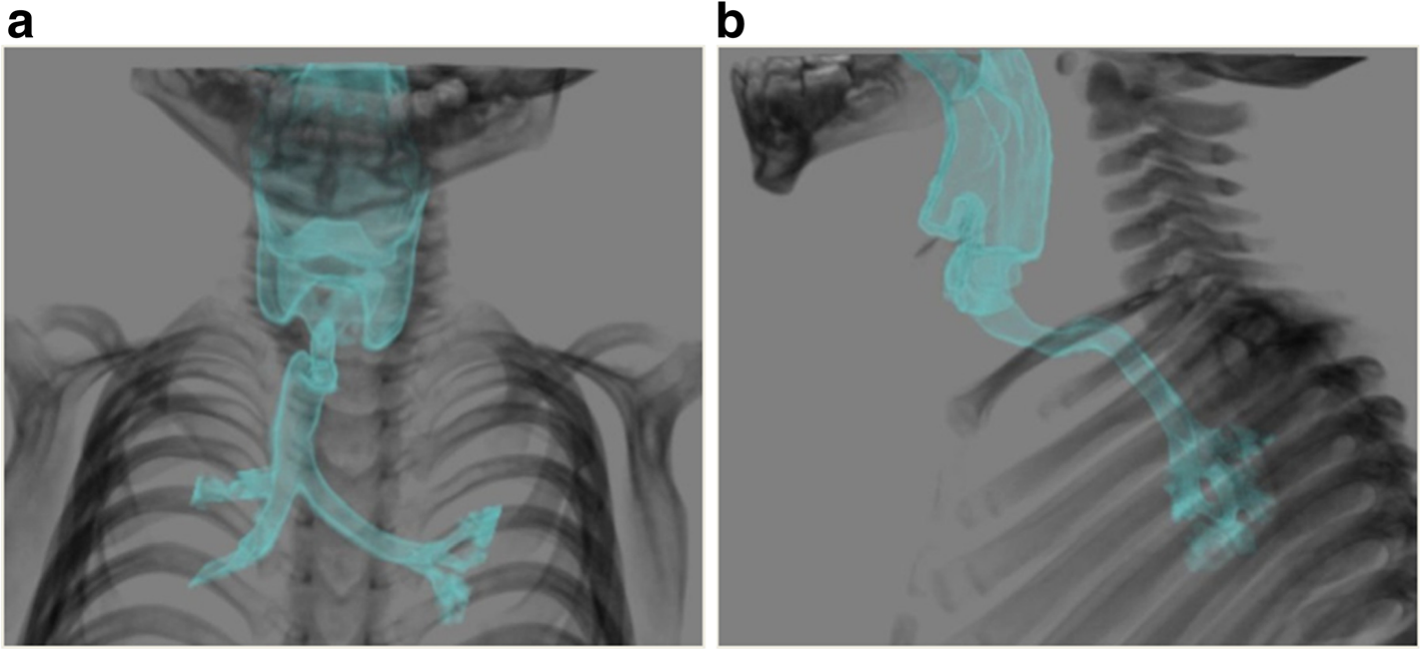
Computed tomography before operation. a Frontal view. b Lateral view. The trachea was severely deviated at an angle of 90 ° from the ventral to dorsal side
